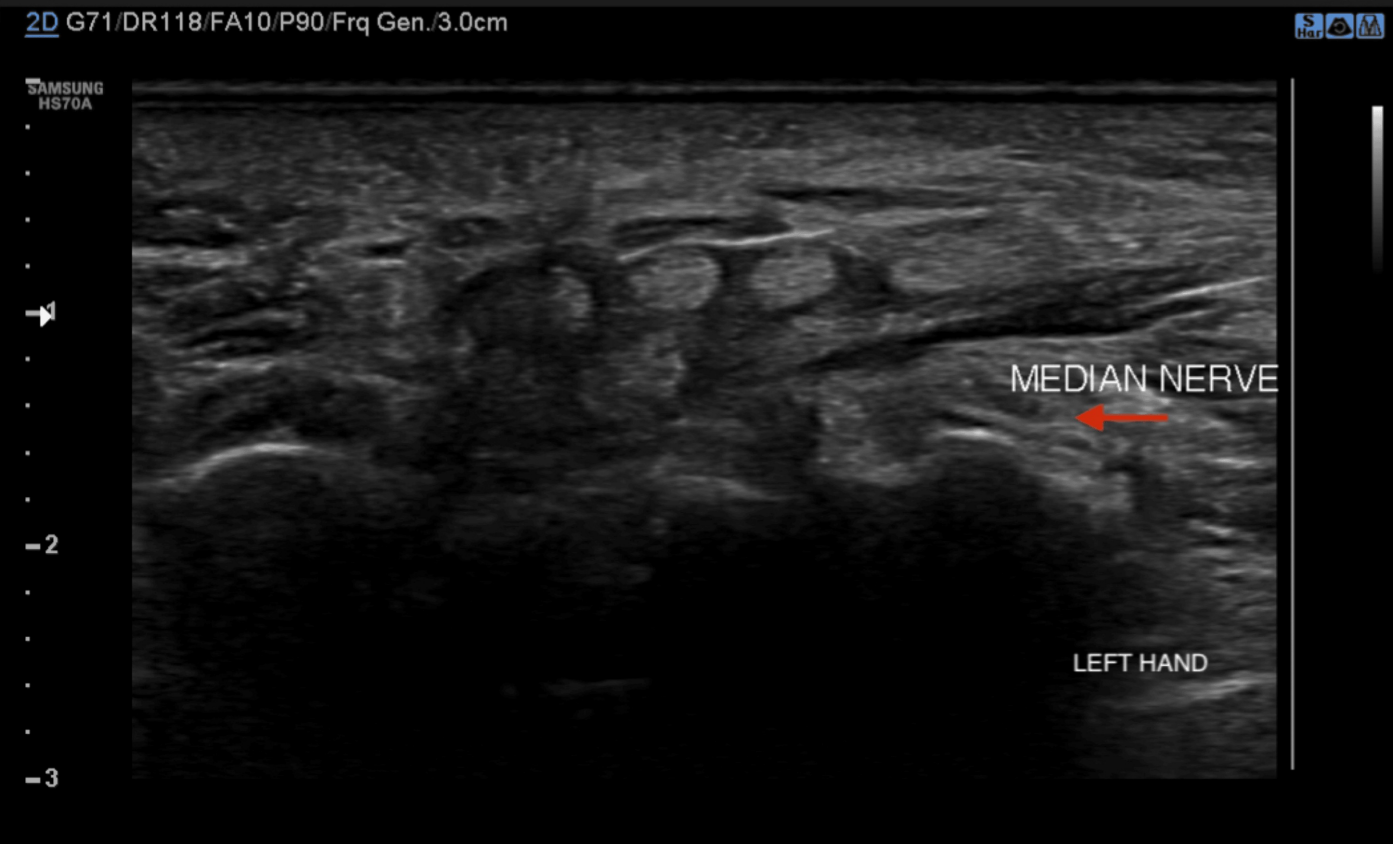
High-resolution ultrasonography of the left median nerve at the wrist High-resolution ultrasonography of the left median nerve showing the median nerve in relation to the flexor retinaculum. Loss of fascicular architecture and echotexture of the nerve with flattening of the nerve is observed with palmar bowing of the flexor retinaculum. Flexor and extensor tendons appear bulky and echogenic with surrounding fluid, indicating the likelihood of tendinitis.
